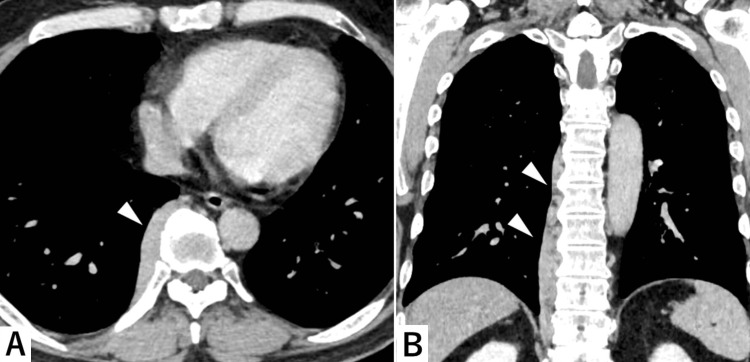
Chest CT scan CT scan (A, coronal view; B, axial view) showing a posterior mediastinal mass adjacent to the 6th-11th thoracic vertebral bodies (arrowheads). CT: computed tomography
